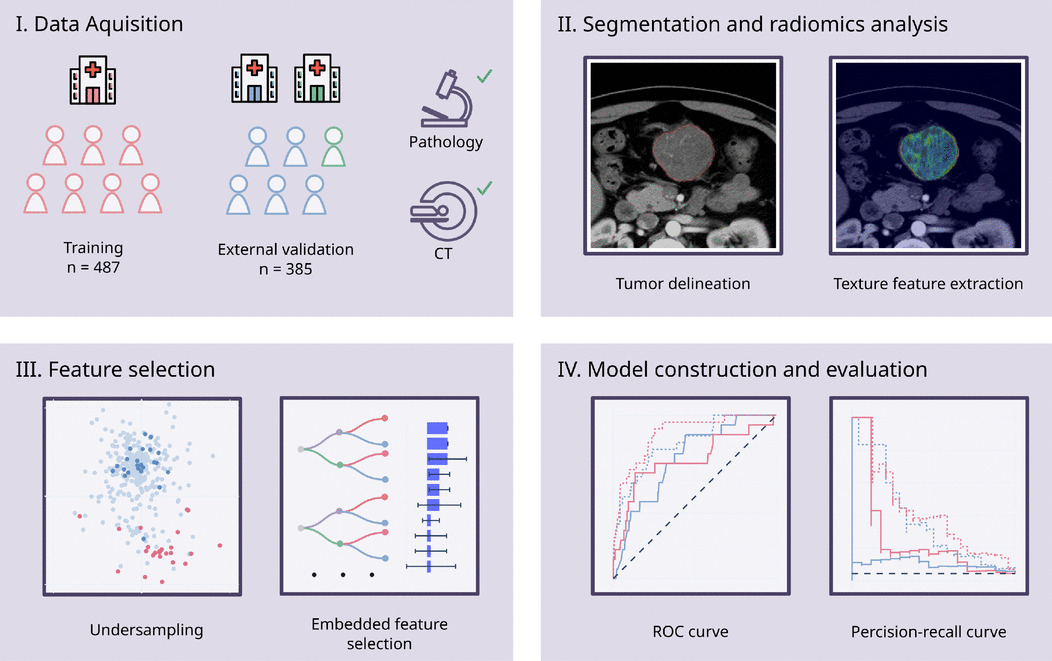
The study design. CT, computed tomography; ROC, relative operating characteristic. Some icons designed by OpenMoji (https://github.com/hfg-gmuend/openmoji) were used under the terms of Creative Commons Share Alike License 4.0 (CC BY-SA 4.0), with modifications to suit our figure requirements
